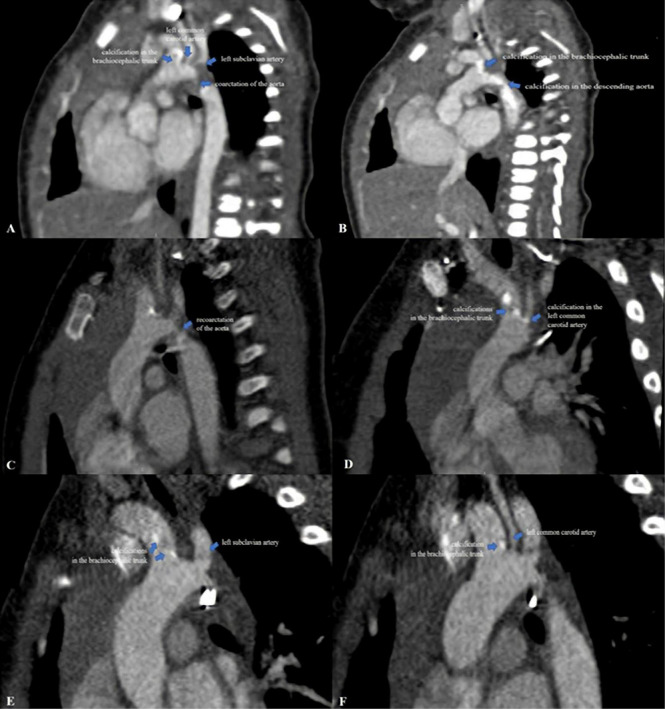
(A,B) Computed tomography images from the neonatal period showing extensive ectopic calcifications in the aortic arch, brachiocephalic trunk, left common carotid artery, left subclavian artery and descending aorta with severe narrowing of the aorta mimicking coarctation of the aorta. Note the brightness of the walls of the aortic arch and descending aorta; (C,D) computed tomography images at 3-months of age showing re-coarctation of the aorta and residual calcifications in the brachiocephalic trunk and left common carotid artery without obstruction; (E,F) computed tomography images at 18-months of age showing the regression of the aortic arch calcifications without stenosis.
